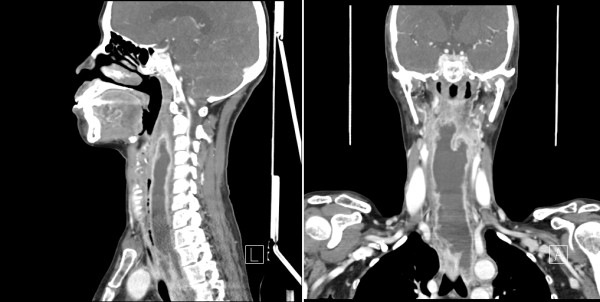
A computed tomography (CT) scan showing a large extended retropharyngeal abscess formation extending to the bronchial bifurcation.
